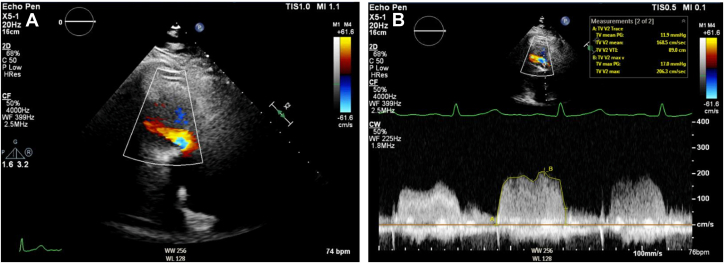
3 Month Follow Up TTE After Initiation of Anticoagulation (A) TTE demonstrating tricuspid inflow with color Doppler jet over the TV. (B) TTE demonstrating no significant improvement in tricuspid inflow velocities despite anticoagulation. TTE = transthoracic echocardiography; TV = tricuspid valve.
